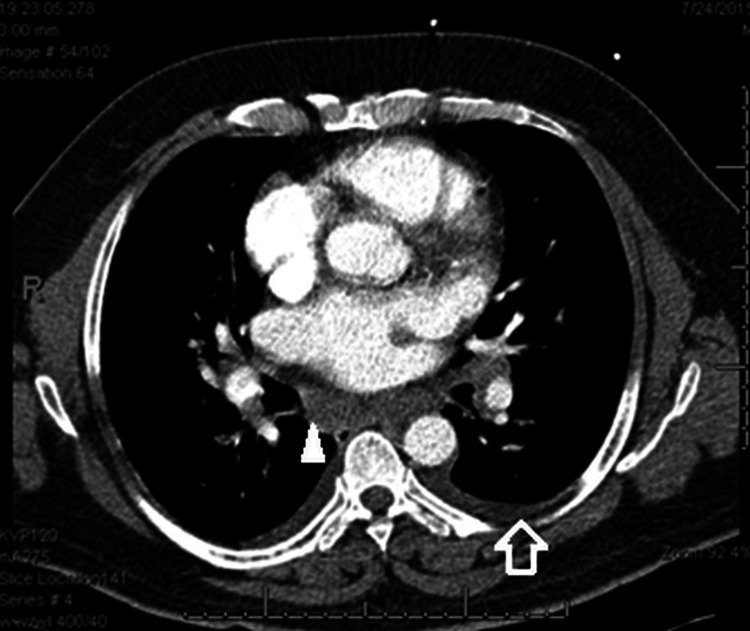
Computed tomography (CT) of the chest showing bilateral pleural effusions (open arrow) and mediastinal adenopathy (arrow head).
